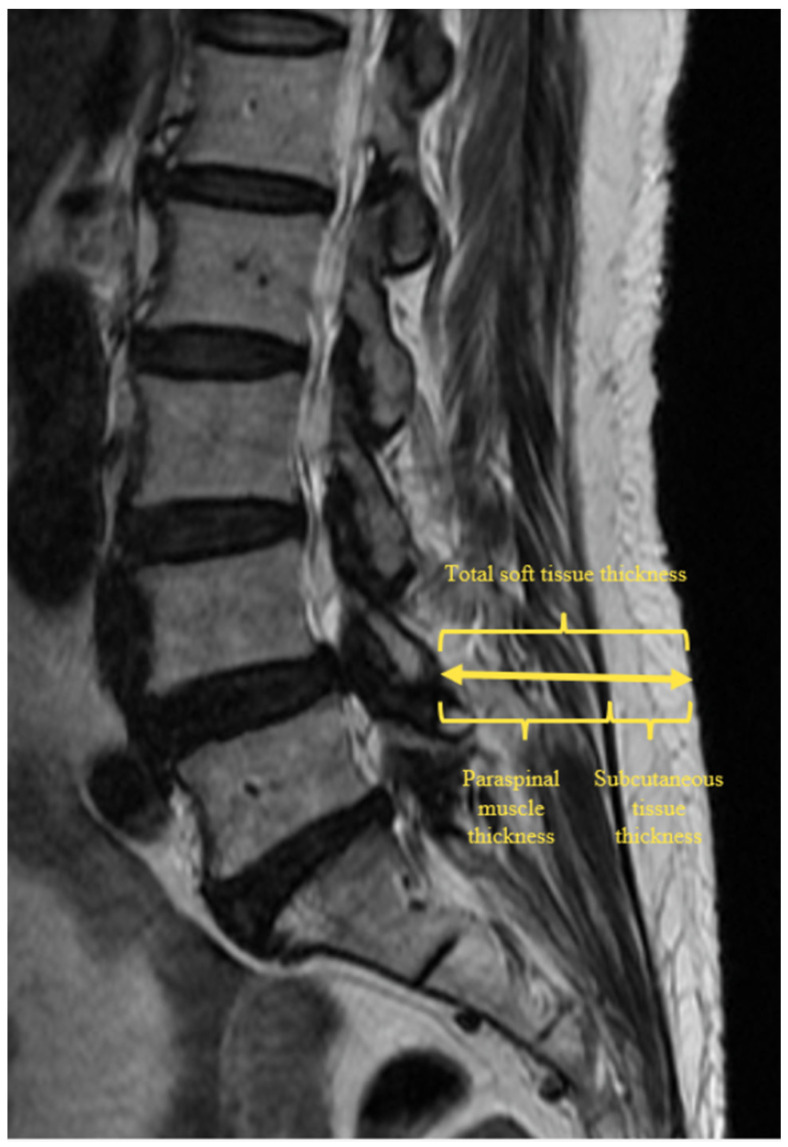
Measurements of total soft tissue, paraspinal muscle, and subcutaneous tissue thickness at the index level on parasagittal MRI of the operative side. MRI, magnetic resonance imaging.
